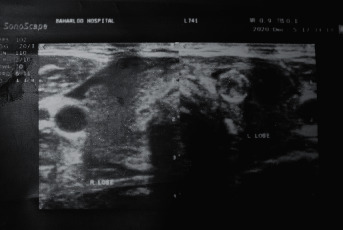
Thyroid ultrasound findings.
